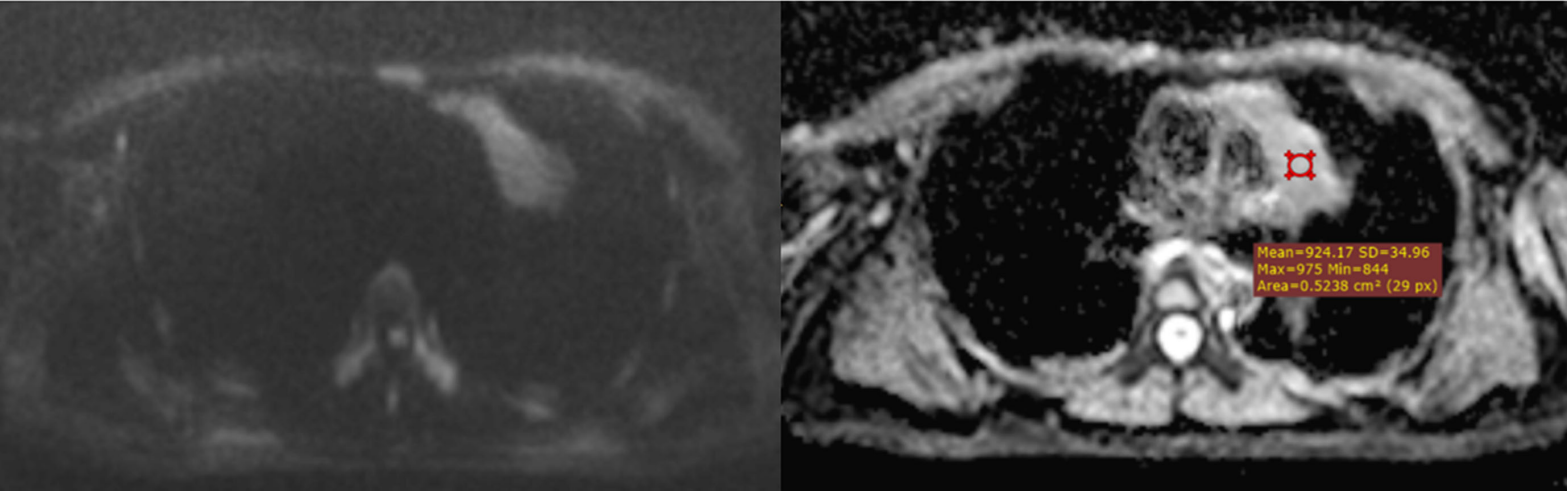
A type B3 thymoma, correctly predicted as a high-risk thymoma on DWI/ADC map. The mean of 3 ADC values obtained by using the hot-spot-ROI-based approach was 0.91 x 10-3 mm2/sec, which was lower than the cutoff of 0.99 x 10-3 mm2/sec.
